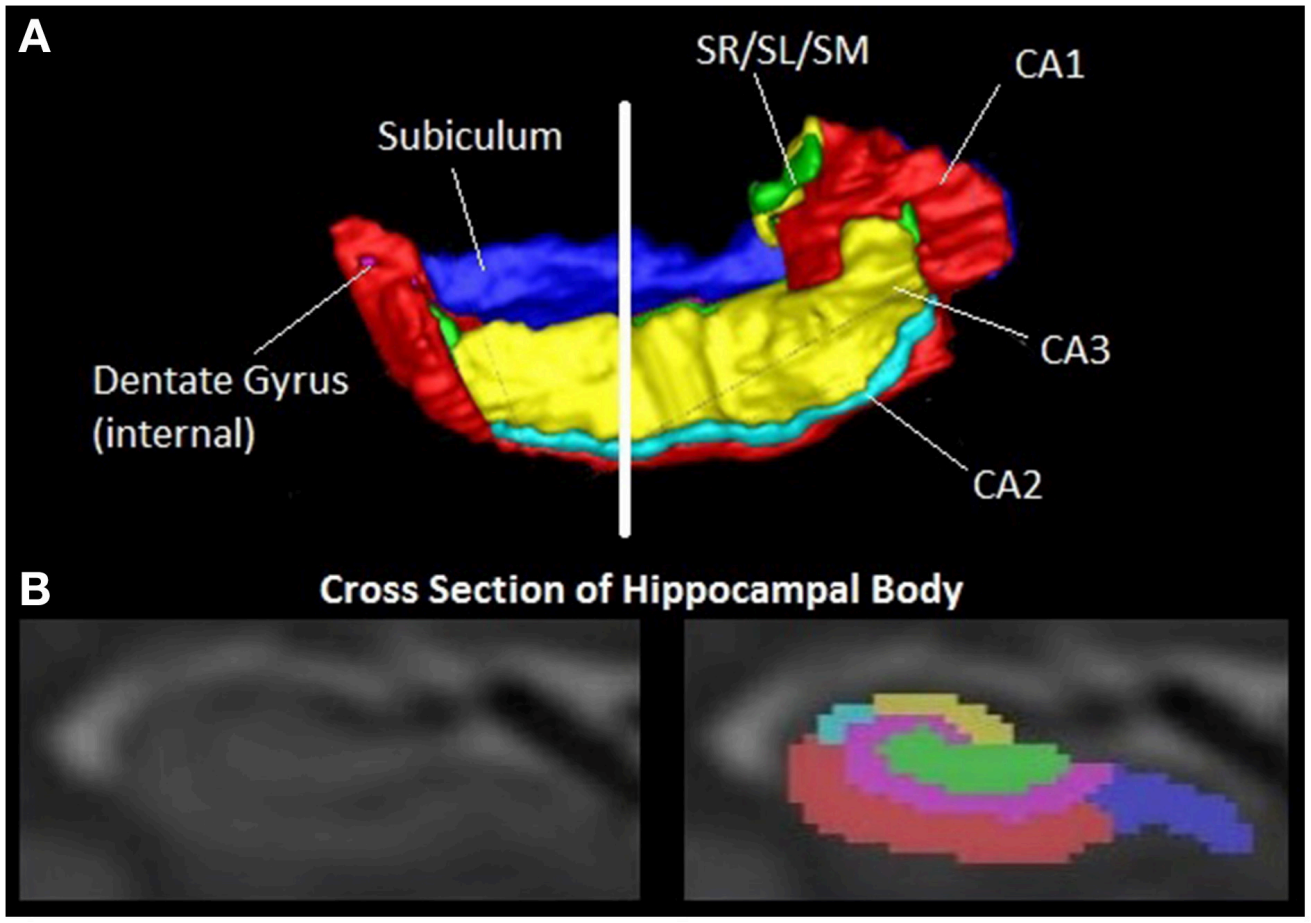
The human hippocampus. Image shows a 3D representation of the right hippocampus with six subfields and a coronal MR image of the hippocampal body with corresponding subfields. Segmentation as described in (Wood et al., 2015). Red = CA1, Light blue = CA2, yellow = CA3, blue = subiculum, pink = dentate gyrus, green = Stratum radiatum/stratum lacunosum/ stratum moleculare (SR/SL/SM).
